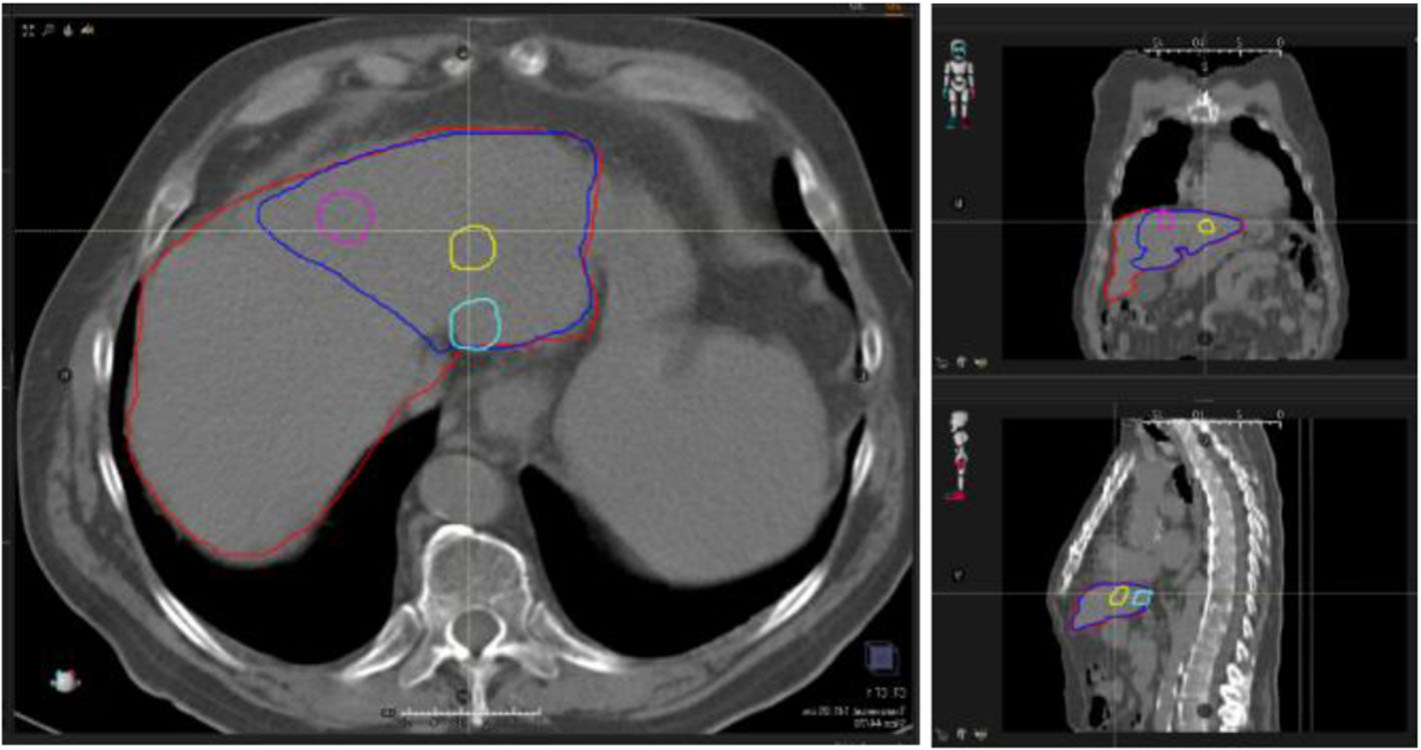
Volumes of interest contoured on the ^99m^Tc-MAA SPECT-CT images used for the pre-therapy dosimetry assessment corresponding to patient 12: whole liver volume delimited by red line, target volume delimited by blue line and individual tumors delimited by pink, yellow, and light blue lines
